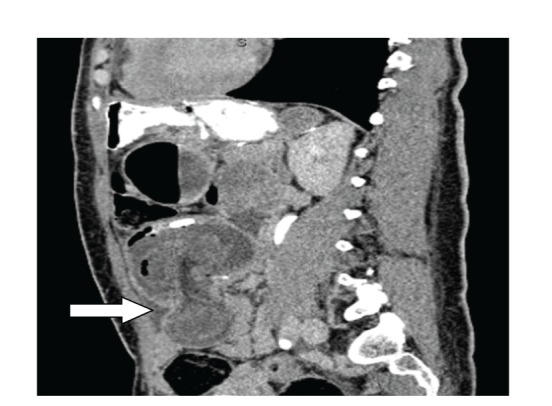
Sagittal view of the CT scan showing intussusception (white arrow—site of intussusception).
